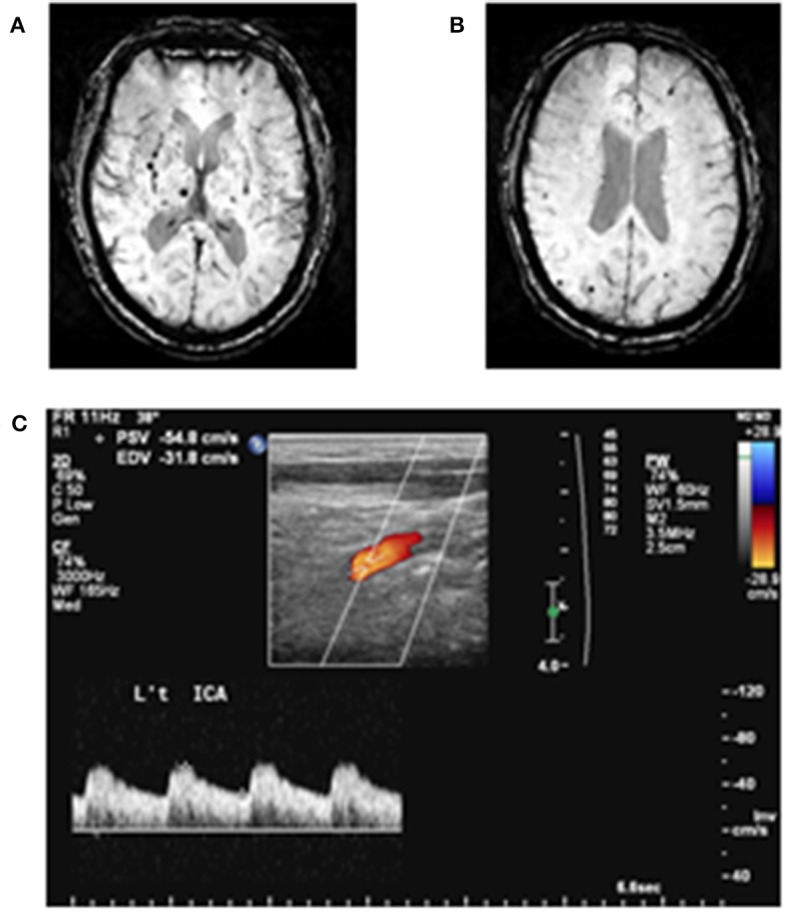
Examples of cerebral microbleeds and arterial pulsatility index measurement. (A) Deep cerebral microbleeds and (B) lobar cerebral microbleeds are demonstrated. (C) Doppler spectrum of internal carotid artery. Arterial pulsatility index is defined as [(peak systolic flow velocity (PSV)—end-diastolic flow velocity (EDV))/mean flow velocity].
